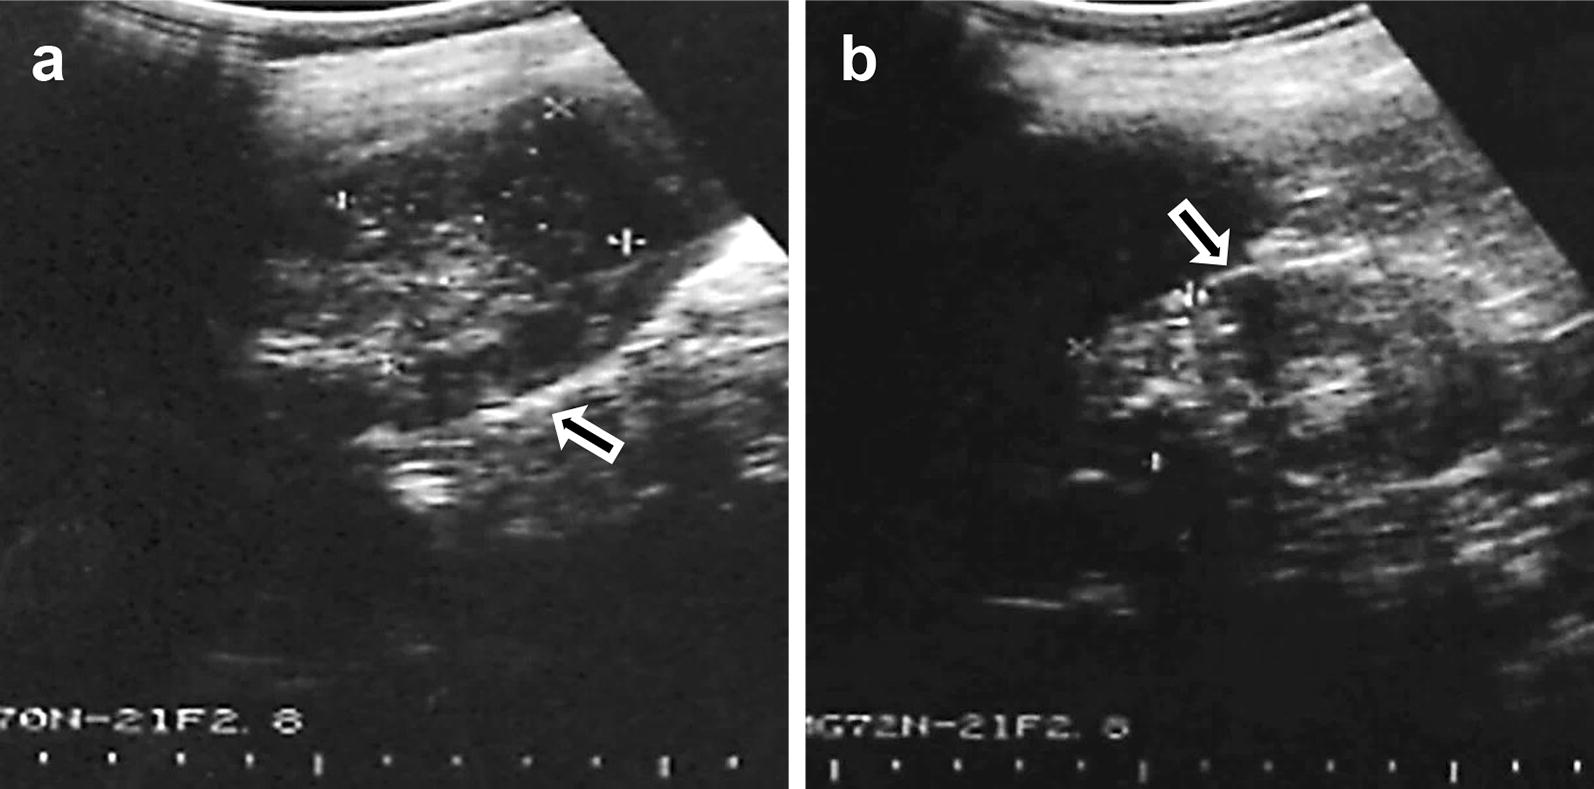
Ultrasonogram of a 48-year-old herdsman who might be concomitantly infected with cystic echinococcosis (CE) and alveolar echinococcosis (AE). a Ultrasonographic (US) examination revealing a 42 × 60 mm sized cystic lesion with diffuse internal calcification and hyperechoic indistinct margin (arrow) on the right hepatic lobe (CE5 stage). b Another view of the US scan showing a 74 × 50 mm sized cystic mass with clusters of multiple echogenic small nodules and a calcified margin (arrow) on the right hepatic lobe (early AE stage)
